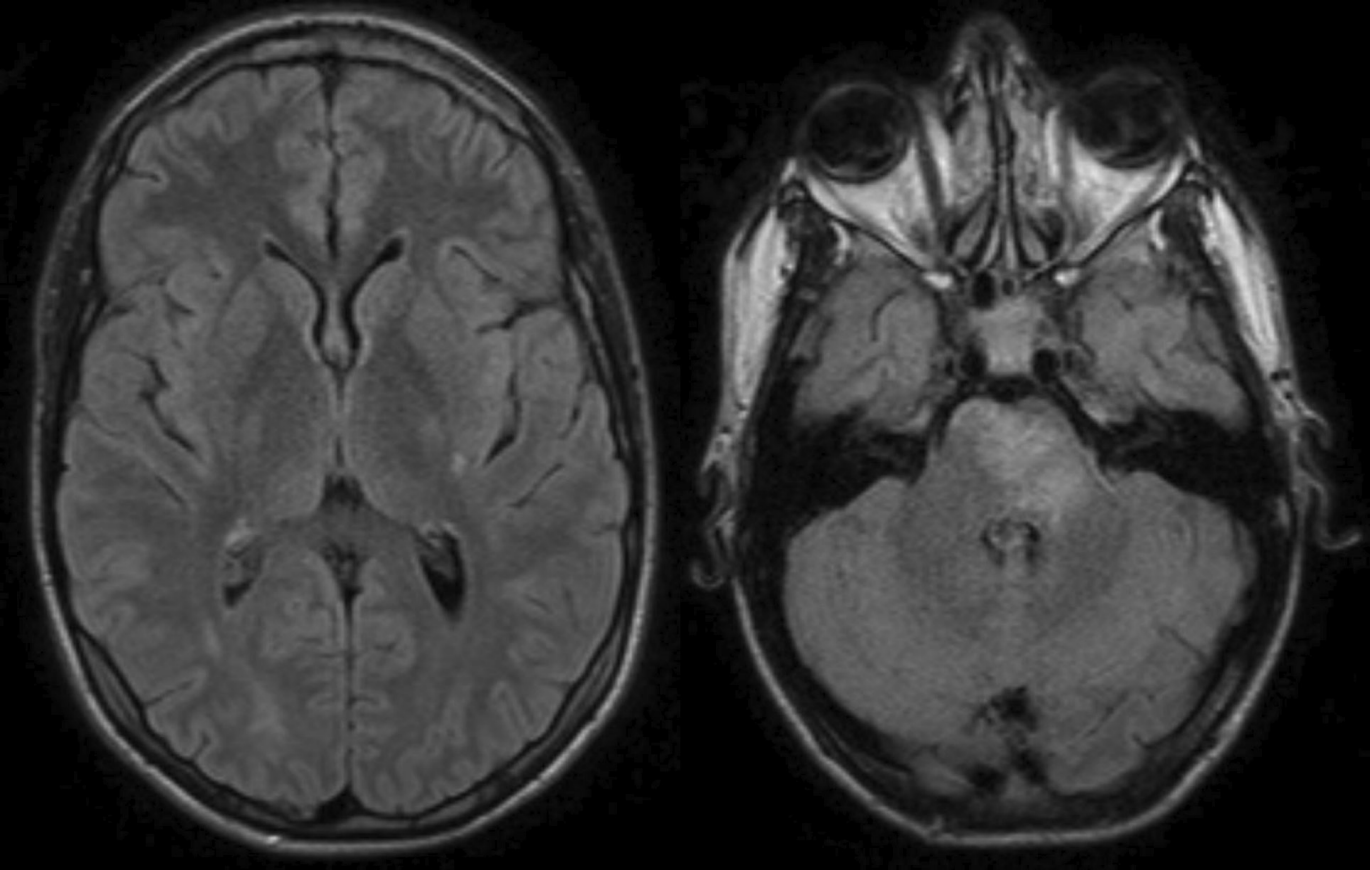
Brain Magnetic Resonance Imaging displaying a hyperintense lesion in the protuberance, the posterior limb of the left internal capsule, and the homolateral fronto‐insular subcortical region in T2 and FLAIR sequences, compatible with vasogenic edema in resolution.
